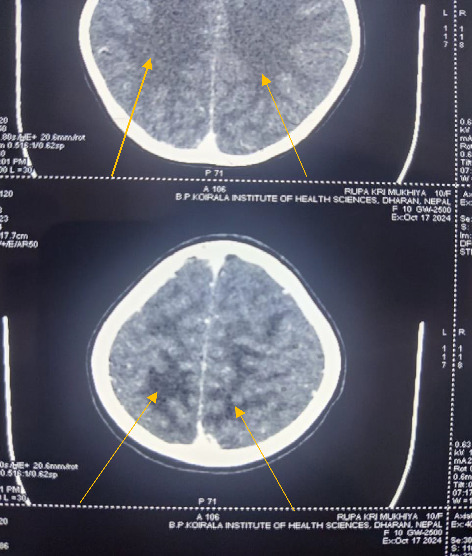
Computed tomography (CT) scan of the head region showing bilateral hypodensities (edema) in the parieto-occipital white matter (indicated by yellow arrow).
